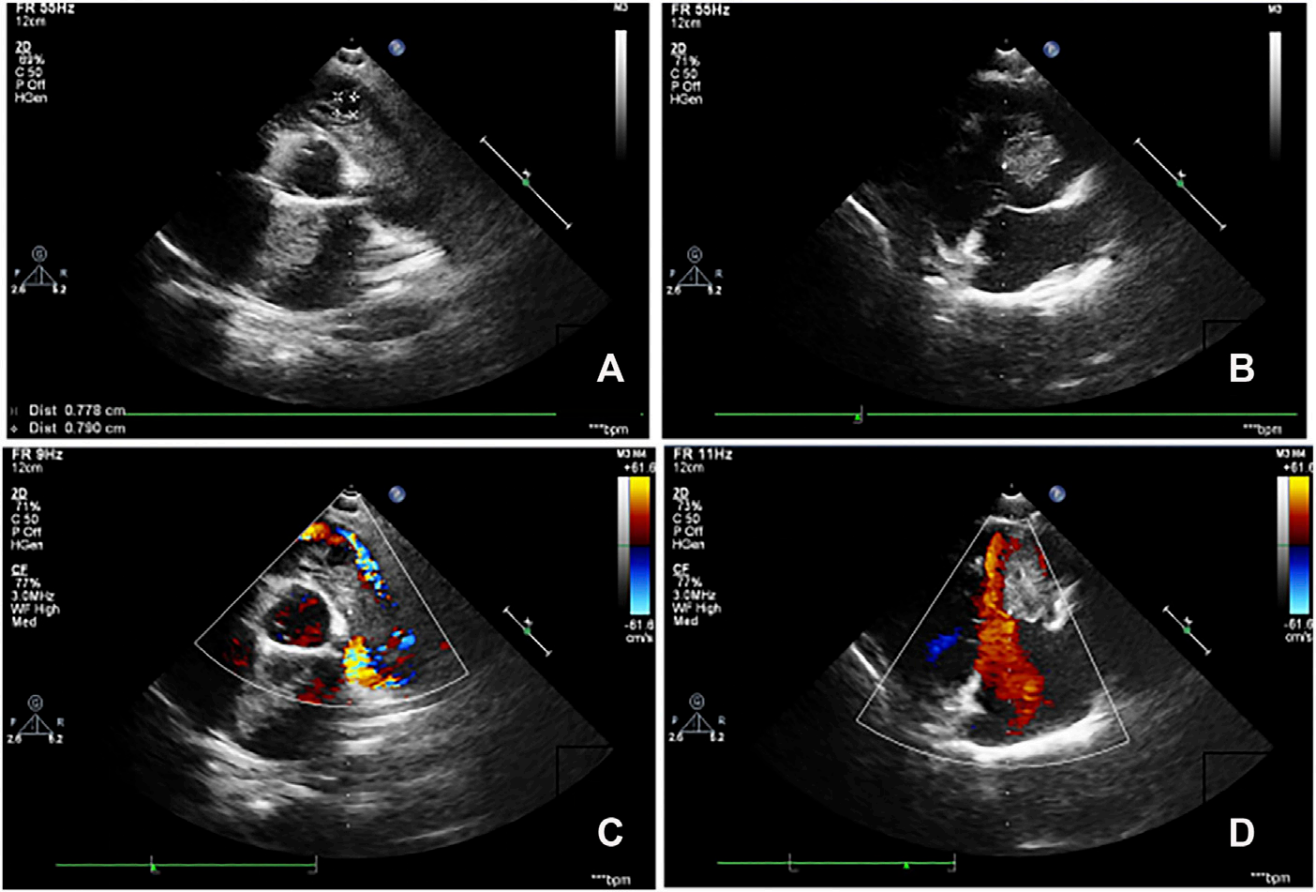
Echocardiography images of the proband. (A,B). Clumpy echogenic mass in the left atrium and right ventricle. (C,D). No obvious enhancement was observed on the enhanced scan.
